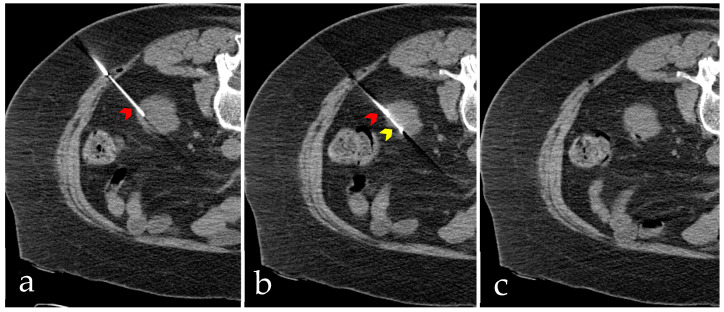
Example of a percutaneous non-target renal biopsy performed under computed tomography (CT) guidance. A 61-year-old man with a known diagnosis of lymphoma developed new-onset proteinuria and was subsequently referred to interventional radiology for the biopsy. The patient had a body mass index of 37.5 kg/m^2^, and the measured distance from the skin surface to the renal parenchyma was 8.5 cm. (a) Axial non-contrast CT image showing a 17-gauge coaxial introducer (red arrowhead). (b) Axial non-contrast CT image showing an 18-gauge core biopsy needle (yellow arrowhead) through the coaxial introducer (red arrowhead) utilizing a cortical tangential approach to sample the renal cortex. A total of four core tissue samples were obtained. (c) Post-procedural non-contrast CT image showing no radiologic evidence of hemorrhagic complication.
